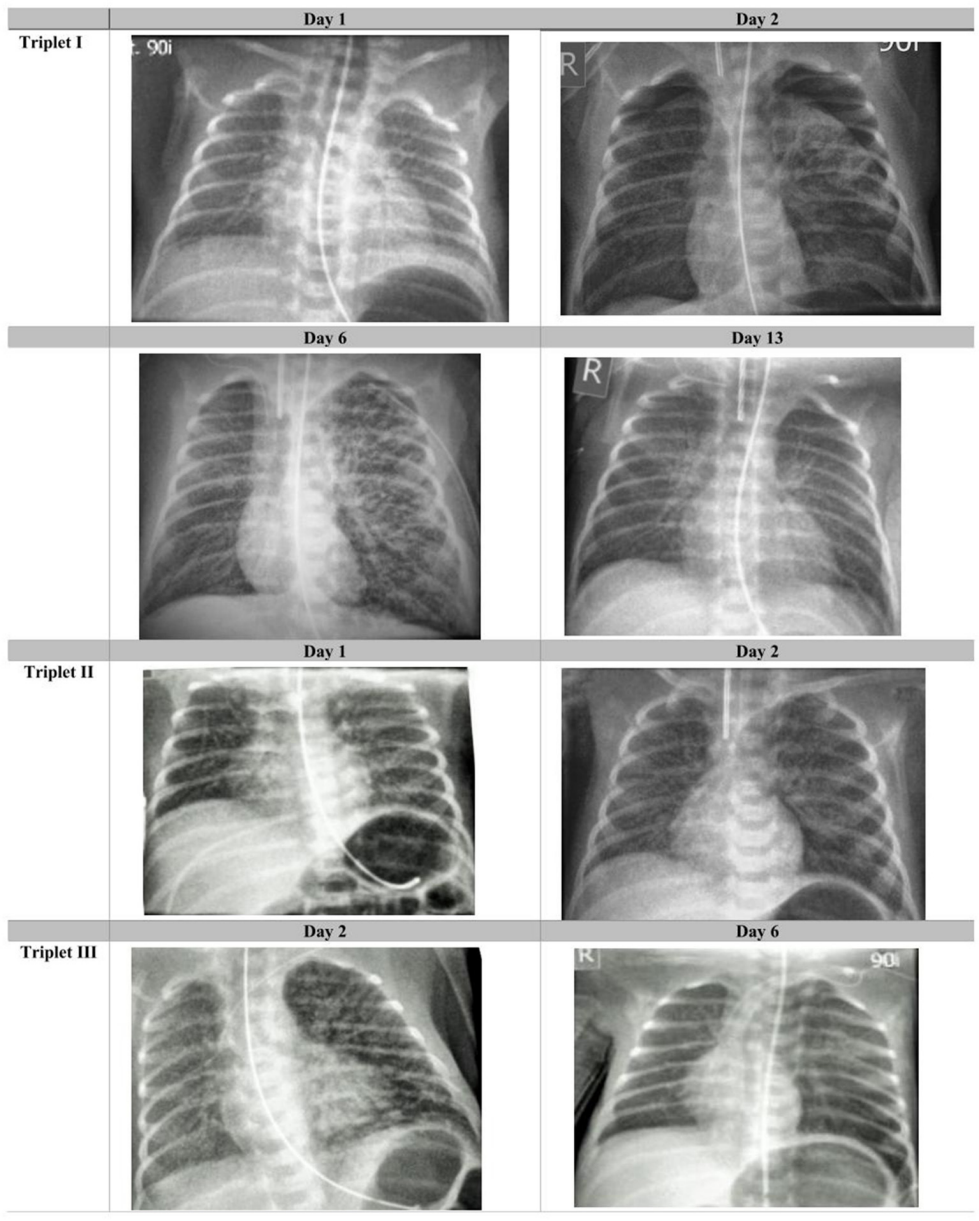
Radiological course in the triplets: Chest radiographies taken on days 1, 2, 6, and 13 after birth in triplet I; on days 1 and 2 in triplet II and on days 2 and 6 in triplet III (selected radiographs shown). Images are windowed in order to optimize visualization of the relevant findings.
